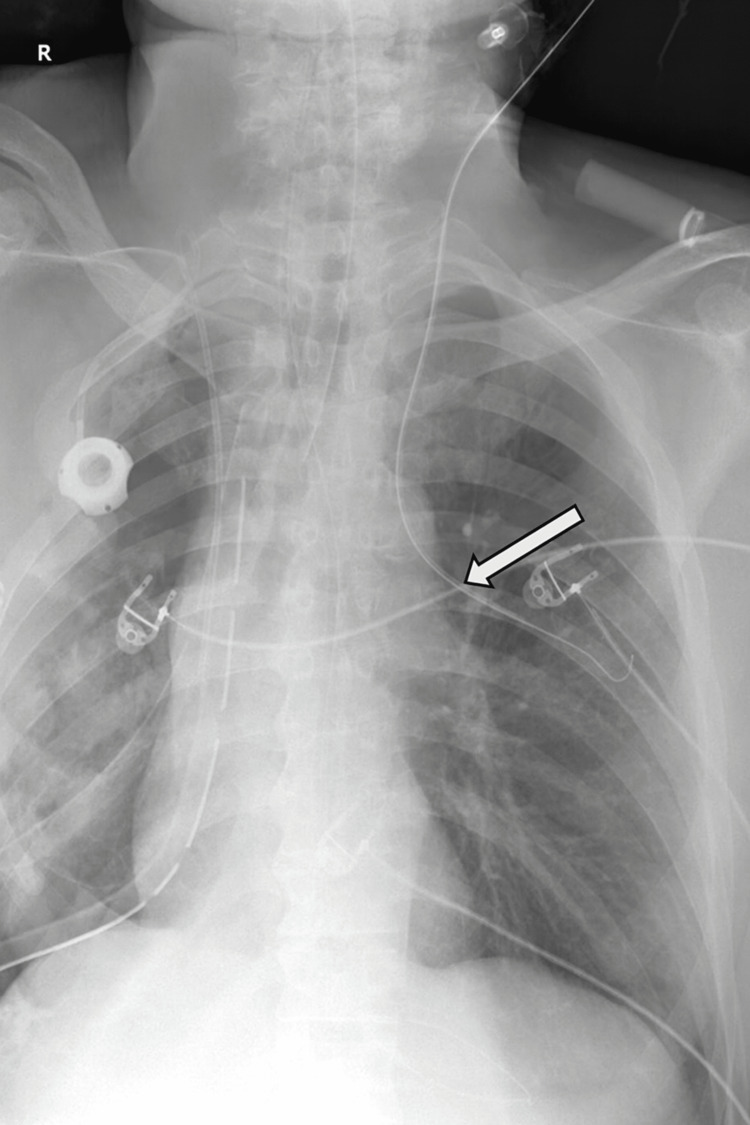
Postoperative chest X-ray demonstrating the central venous catheter positioned within an anomalous vascular structure (arrow), later identified as a left-sided partial anomalous pulmonary venous connection. The catheter tip appears unusually high and lateral, raising suspicion for malposition and prompting further investigation with computed tomography angiography.
